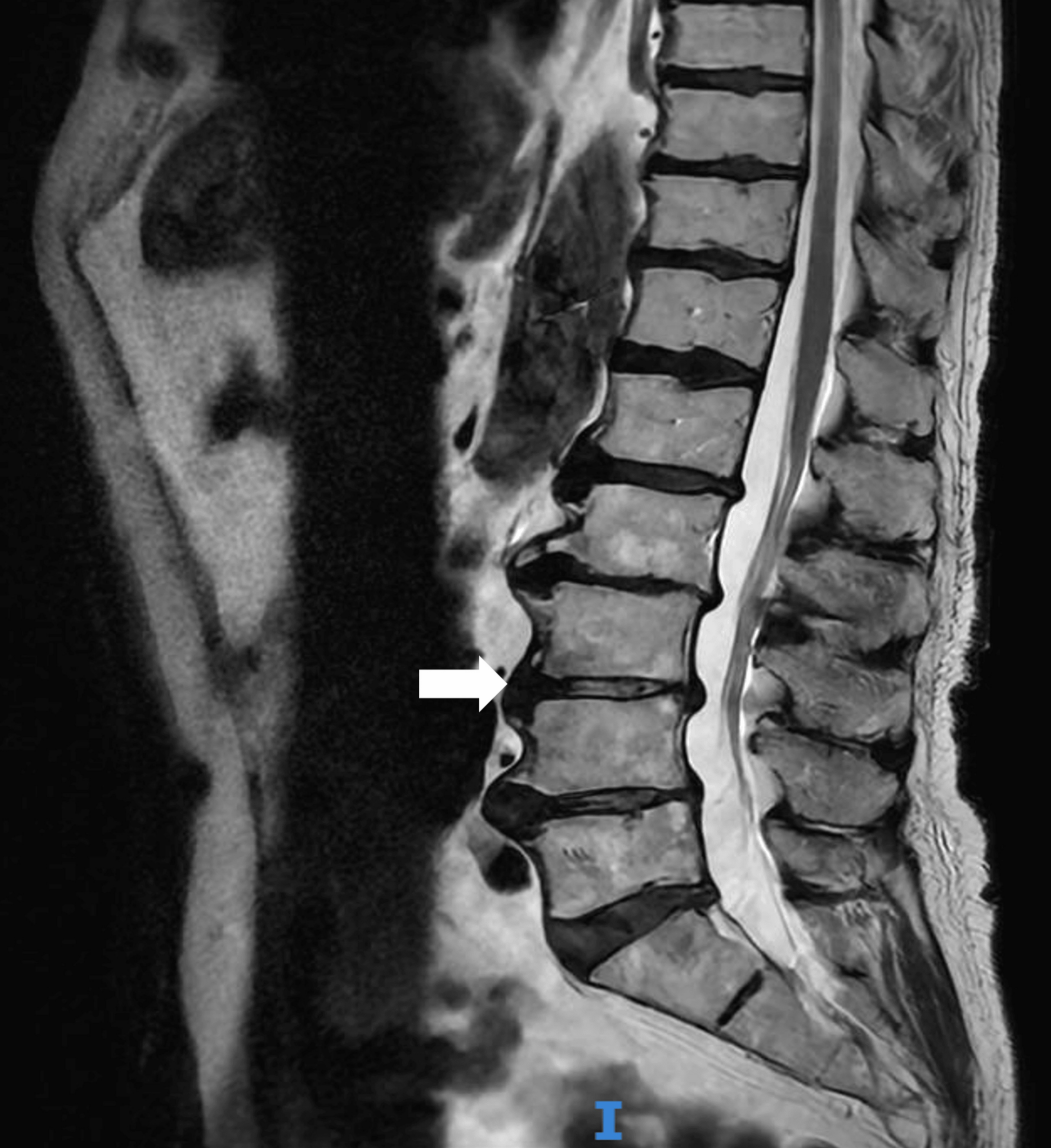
Sagittal T2-weighted MRI lumbar sacral spine Shows multilevel disc bulge extending from L1 to S1 with multiple impinged nerves, most significant at L3/L4.
